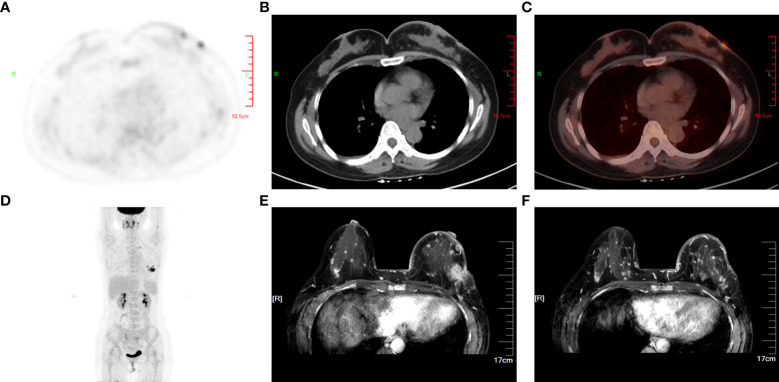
Primary lesion and invasive metastasis in the breast. PET/CT (A–D) revealed multiple masses with increased glucose metabolism in the lateral quadrant of the left breast as well as nodules with slightly increased glucose metabolism in the lateral quadrant of the right breast, which indicated aggressive invasion of the primary tumor on the left. MRI (E, F) in axial sections of the patient’s breast before chemotherapy showed the primary lesion in the left breast and multiple contralateral breast metastases.
